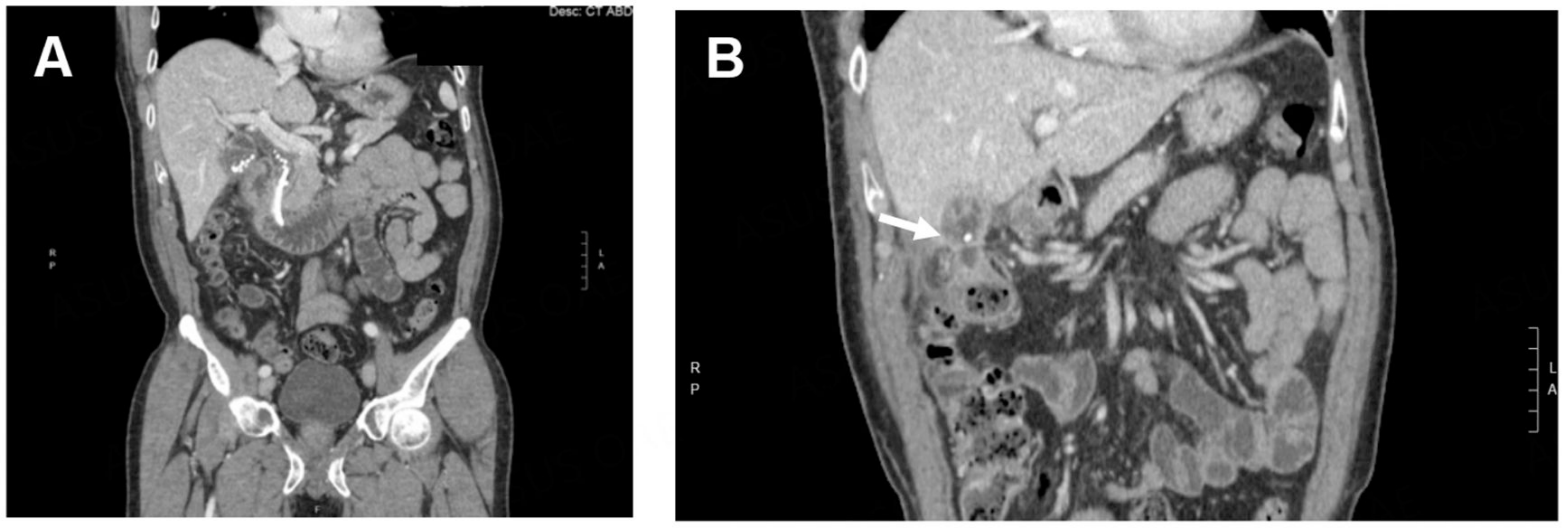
(A) Gallstones in the gallbladder and common bile duct and a stent are observed on a radiograph; (B) a cholecystocolonic fistula is observed (white arrow) on a radiograph.
